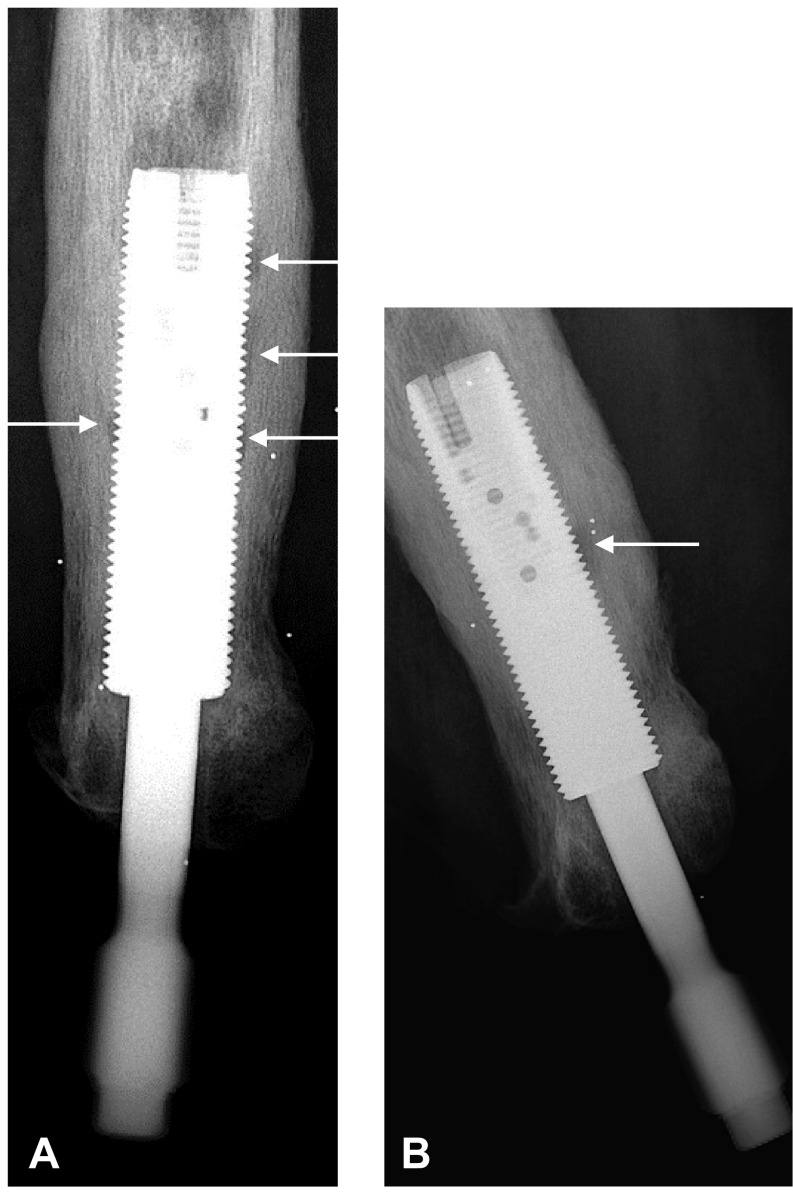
(A) AP and (B) lateral view plain radiographs show small zones of radiolucency (arrows) between the implant and bone in a male patient with osteomyelitis around an osseointegrated implant in the left femur. Free projection of the implant threads is important for correct evaluation.
